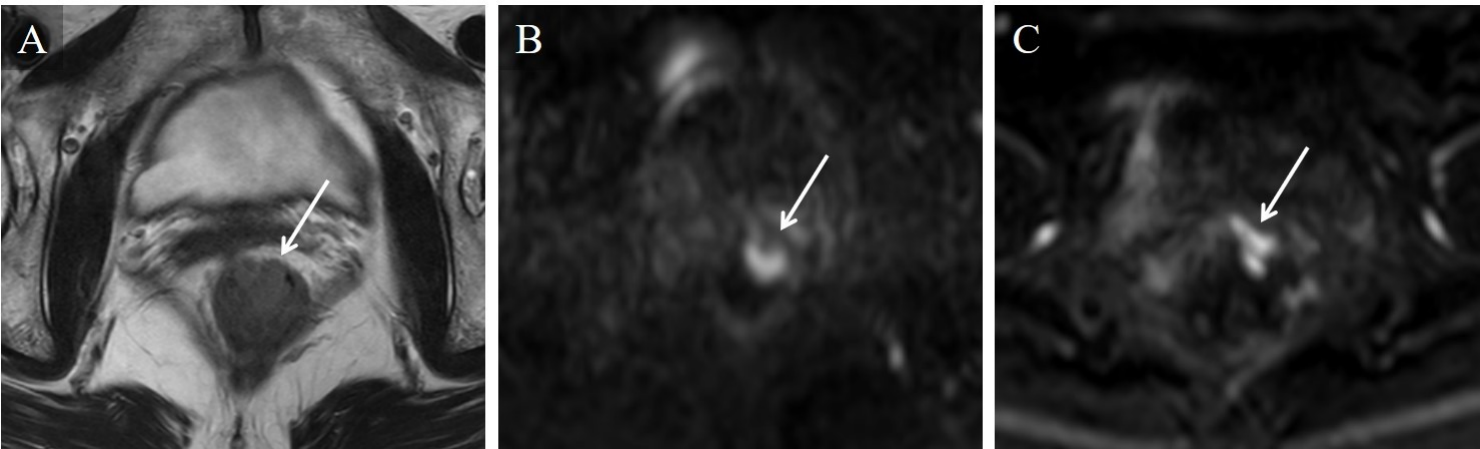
Stage T1 rectal carcinoma in a 75-year-old woman. From the T2WI image (A) alone, all readers misdiagnosed the stage. On the TSE-DWI image (B), an area of high signal intensity with a low signal intensity of stalk was depicted and diagnosed as stage T1. However, this finding was not presented on the EPI-DWI image (C) due to distortion artifacts, and all readers misdiagnosed the stage, as with the T2WI image alone.
